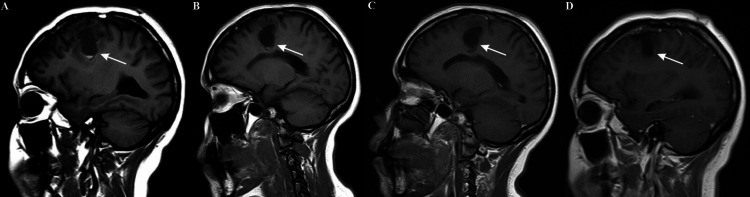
Brain magnetic resonance imaging during the follow-up Sagittal view of T1-weighted sequences with contrast at two months (A), three months (B), four months (C), and nine months (D).
